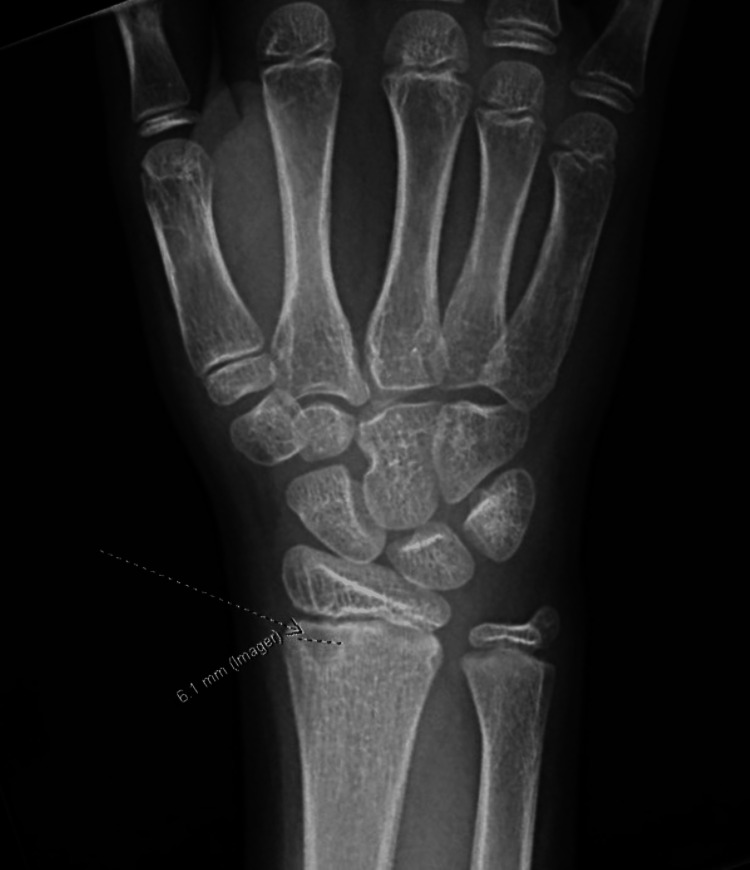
X-ray highlighting a 6.1 mm lytic lesion found on the right distal radius (arrow).
